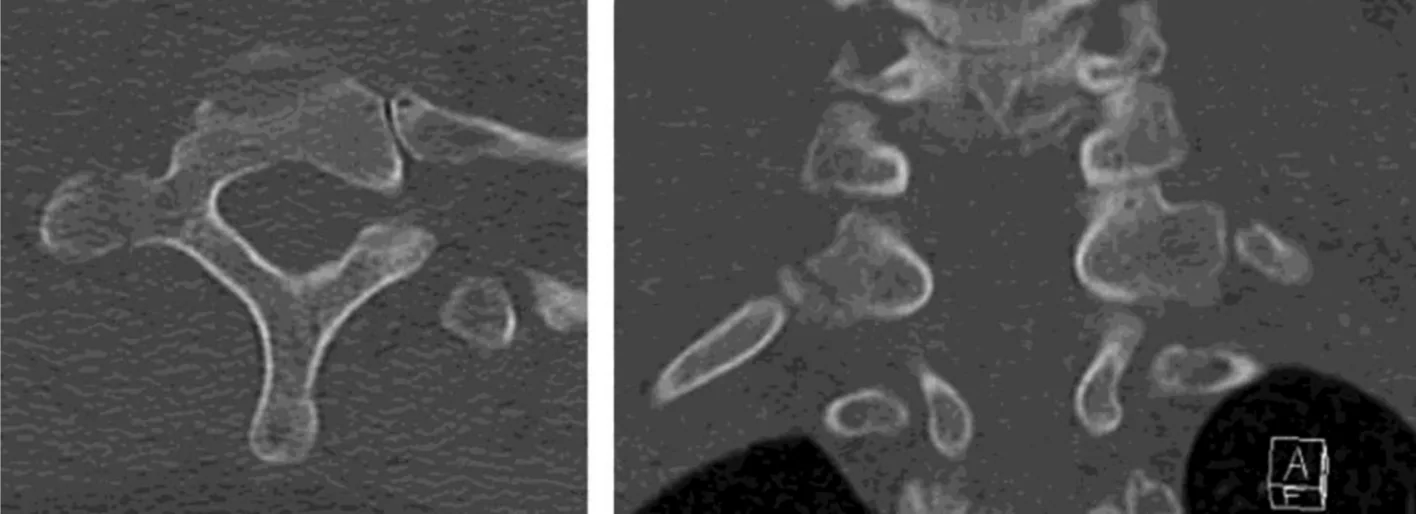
Axial and coronal CT scans of an isolated thoracic transverse process fracture [56]; reproduced from Peterson et al. [56], with permission
